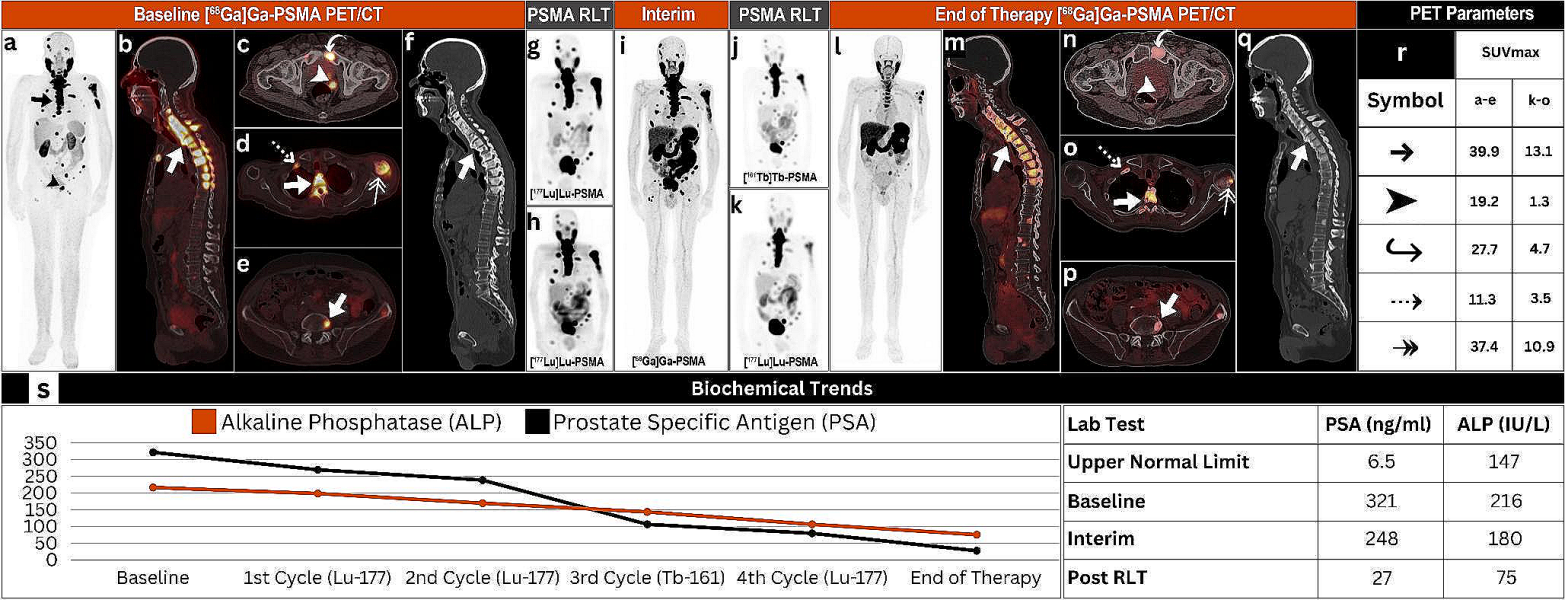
(a-f) Baseline [^68^Ga]Ga-prostate-specific membrane antigen (PSMA) positron emission tomography/computed tomography (PET/CT) demonstrates intense PSMA localization at sites of primary prostatic lesions and skeletal metastases (arrows). (g, h) Two cycles of [^177^Lu]Lu-PSMA, dosed at 7.4 GBq each, were administered with adequate scintigraphic localization. (i) Interim [^68^Ga]Ga-PSMA shows persistent viable disease. (j) A single dose of 5.5 GBq of [^161^Tb]Tb-PSMA followed by (k) 7.4 GBq of [^177^Lu]Lu-PSMA were administered with adequate scintigraphic localization. (l-q) Post-therapeutic [^68^Ga]Ga-PSMA highlighted partial disease control (arrows) (r) with favorable molecular imaging derived maximum standardized uptake values and (s) biochemical metrics achieved
